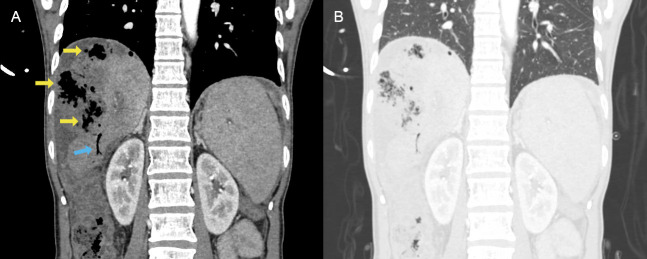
A) A coronal cut of the computed tomography (CT) abdomen. Air is seen within the liver parenchyma in two patterns: large collections of air within the liver masses (yellow arrows), and smaller tracks of air extending to the periphery of the liver (blue arrows). B) Coronal cut of the CT abdomen in the lung preset. The lung bases appear clear.
